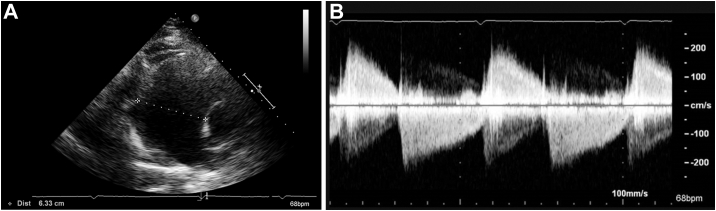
Findings on Transthoracic Echocardiography (A) Image reveals a severely dilated pulmonary artery measuring 6.3 cm. (B) Continuous-wave Doppler shows steep deceleration across the pulmonic valve, consistent with severe pulmonary regurgitation.
